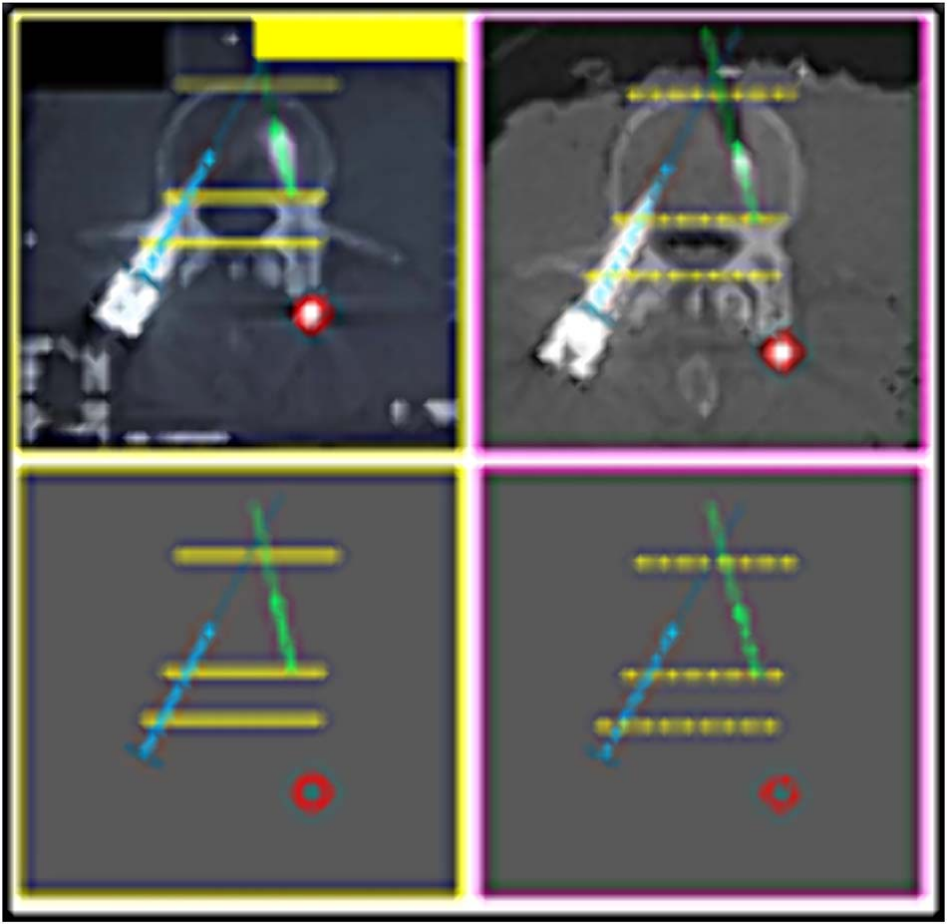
Contour of the L3 vertebral body. Upper panels, comparison between the axial slices of the antemortem (left) and postmortem (right) CT scans. Lower panels, respective concordant templates.
